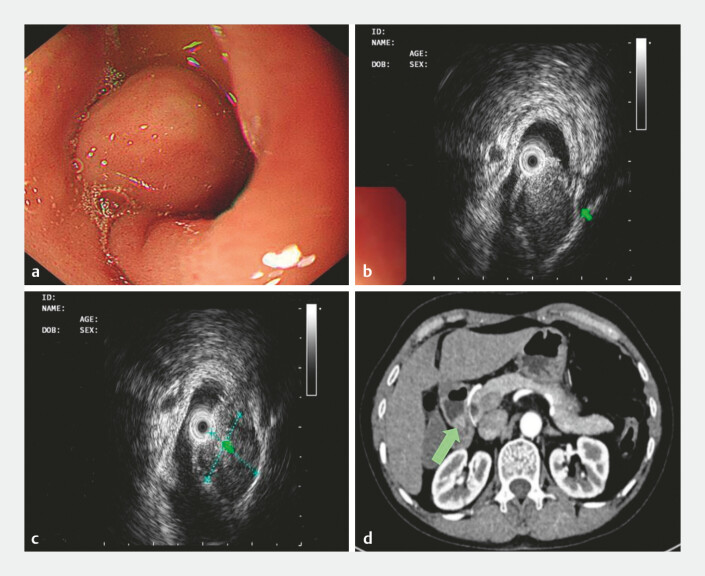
Preoperative endoscopic ultrasonography and contrast-enhanced CT examination results.
